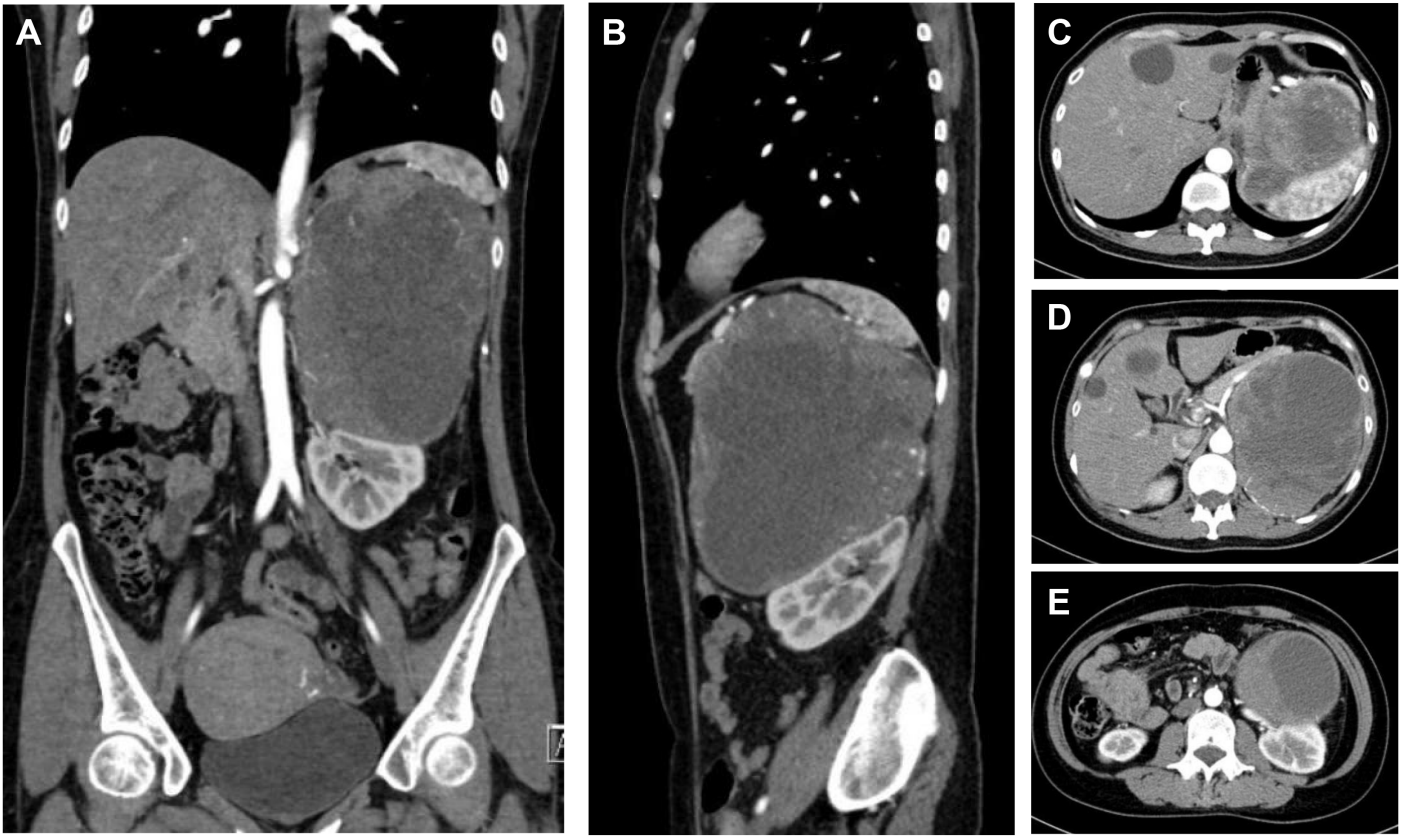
CT of a giant PCC in the left perirenal area. (A) Coronary position of the PCC. A mass of mixed density, with approximately 14.4cm×12.5cm in size, had significantly enhanced solid components. (B) Sagittal position of the PCC. The tumor pushed against the spleen and the left kidney. (C) The upper part of the PCC compressed the stomach. (D) The middle part of the PCC was not well demarcated with the tail of the pancreas and was close to the celiac trunk and the abdominal aorta. (E) The lower part of the PCC pressed on the left kidney and was close to the left renal pedicle.
